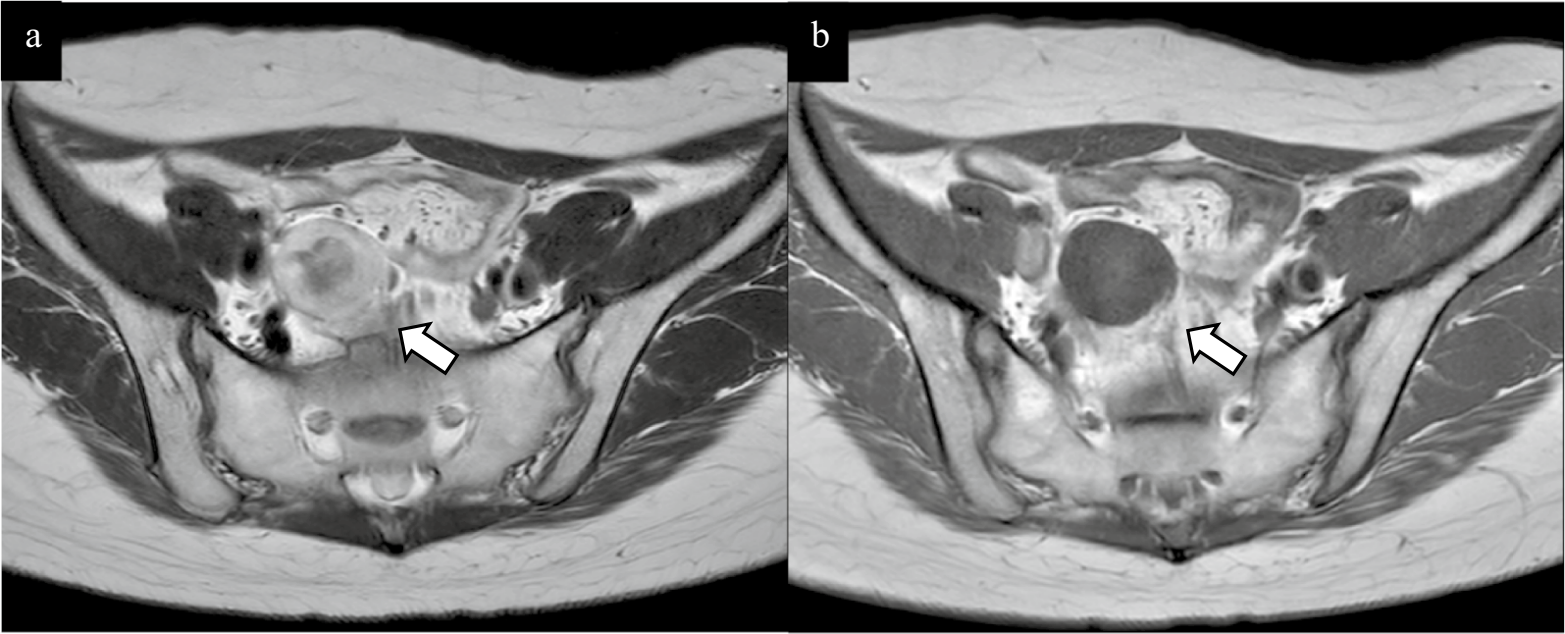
Abdominoopelvic magnetic resonance imaging (MRI). a Axial T2-weighted fast spin-echo MRI showed high signal at the limbus and mildly low signal in the interior. b Axial T1-weighted images showed heterogeneous low signal
